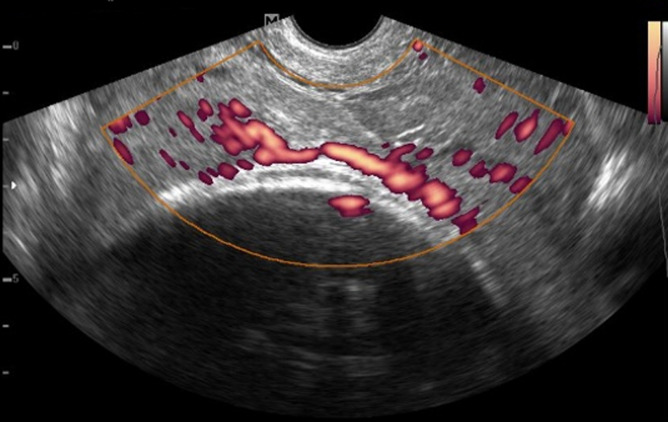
transvaginal ultrasound with power Doppler showing the fetal vessel between a bilobed placenta
